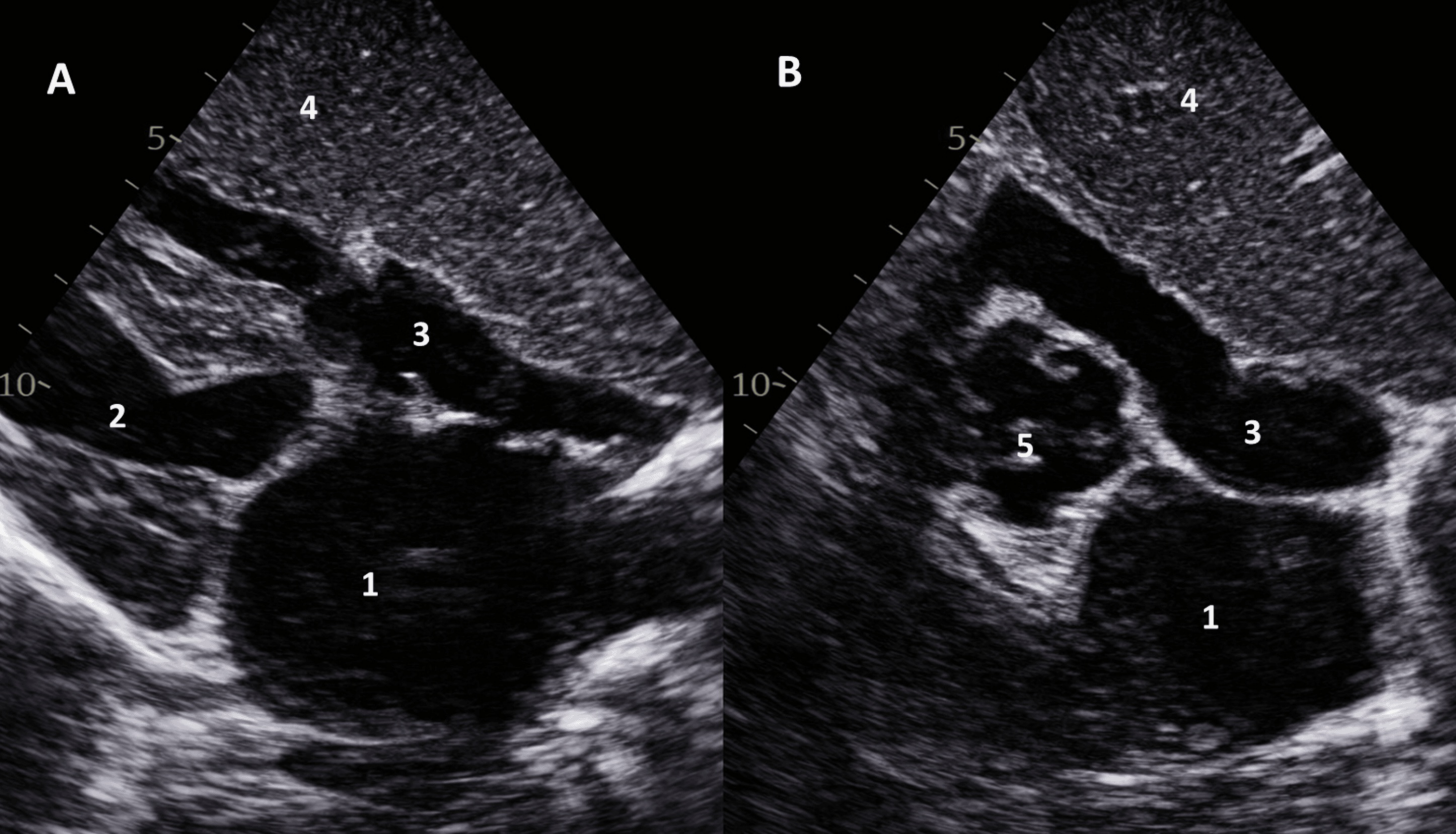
Transthoracic echocardiography (TTE) in the subcostal view demonstrating the liver on the left side. (A) Long-axis view; (B) short-axis view. 1: left atrium; 2: left ventricle; 3: right atrium; 4: liver; 5: aortic valve
